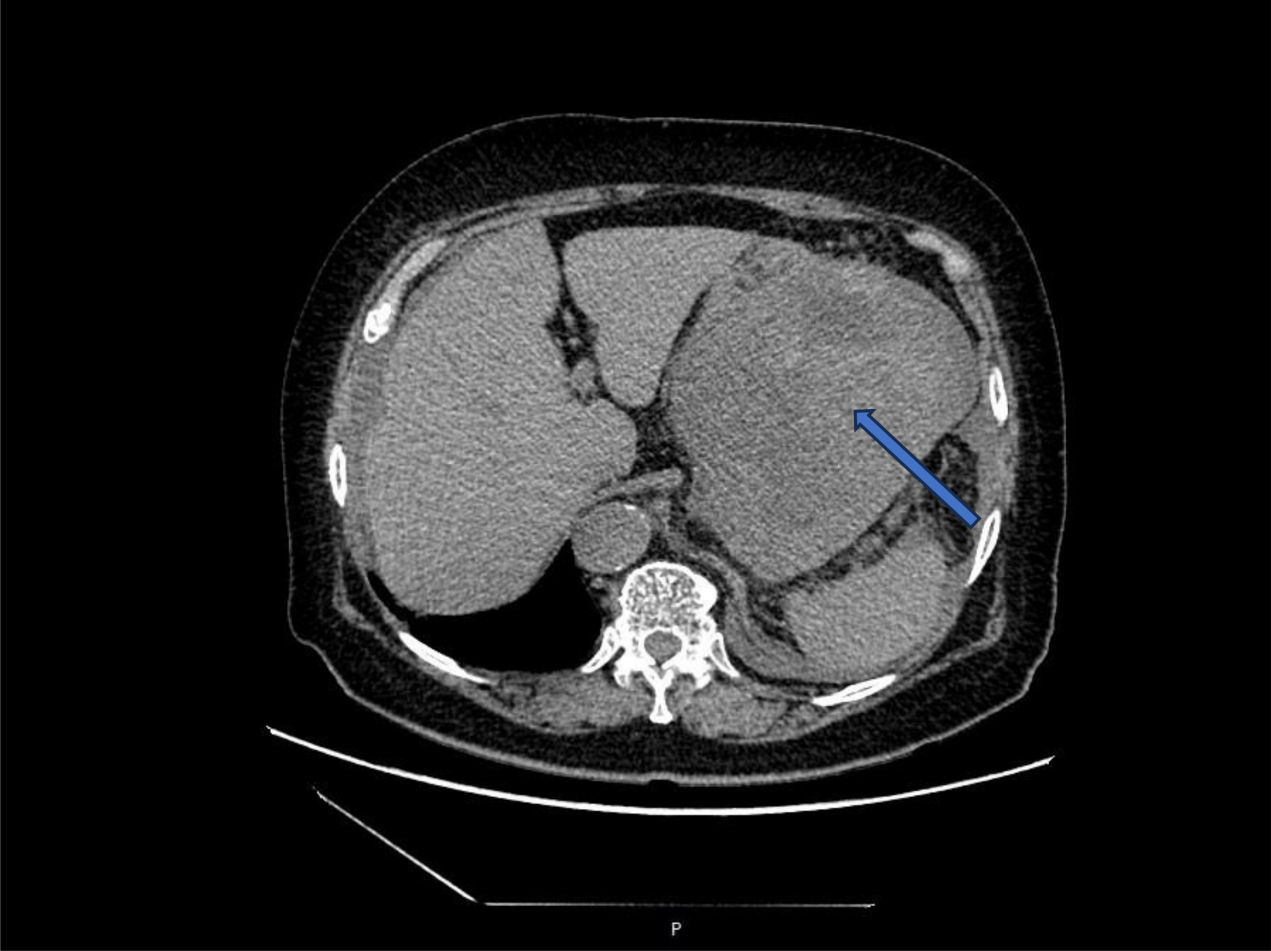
CT of midsection, with the marked areas representing lesions.
